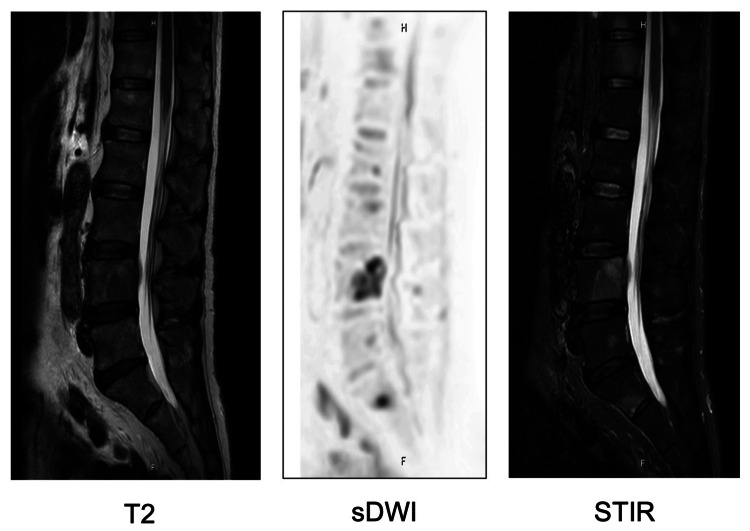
MRI demonstrating osseous metastases in the lumbar spine prior to treatment initiation. sDWI: standard diffusion-weighted imaging; STIR: short tau inversion recovery
